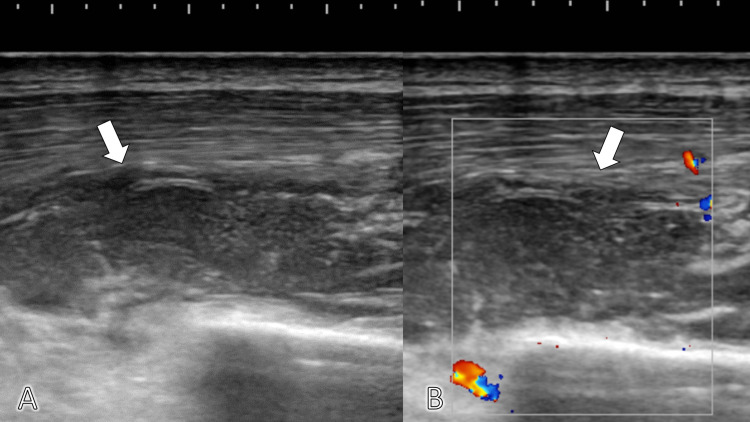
Soft-tissue ultrasonography Soft-tissue ultrasonography of the left anterior chest wall showing (A) fluid retention in the left intercostal muscle (white arrow) and (B) the increase in vascular flow (white arrow; color spotting).
